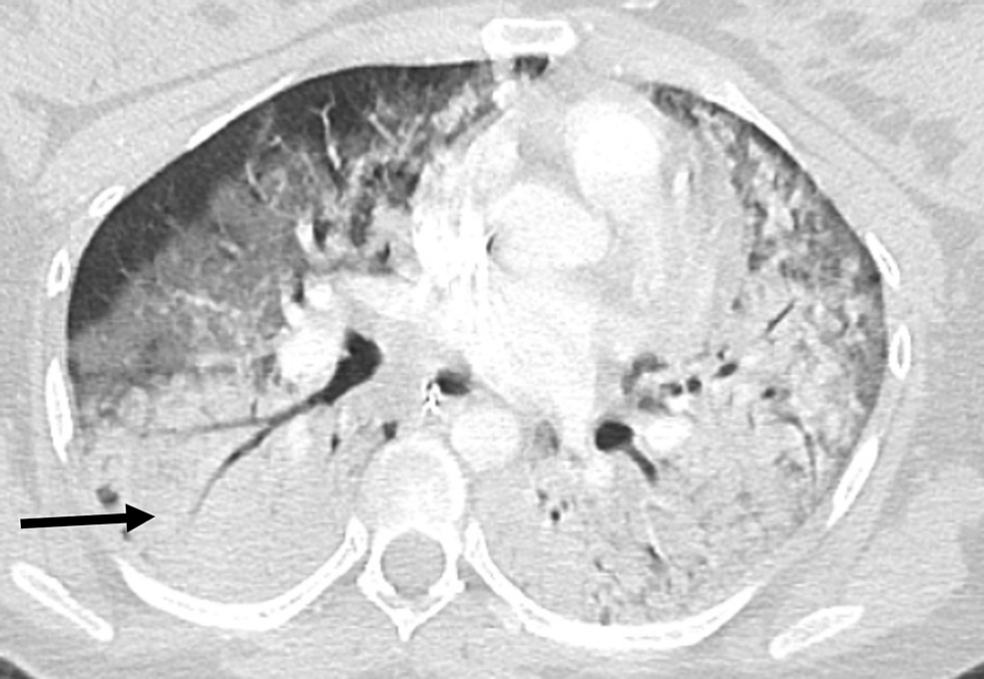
Dense bilateral pulmonary infiltrates on CT scan chest indicating ARDS (as indicated by the arrow) ARDS: Acute respiratory distress syndrome.
